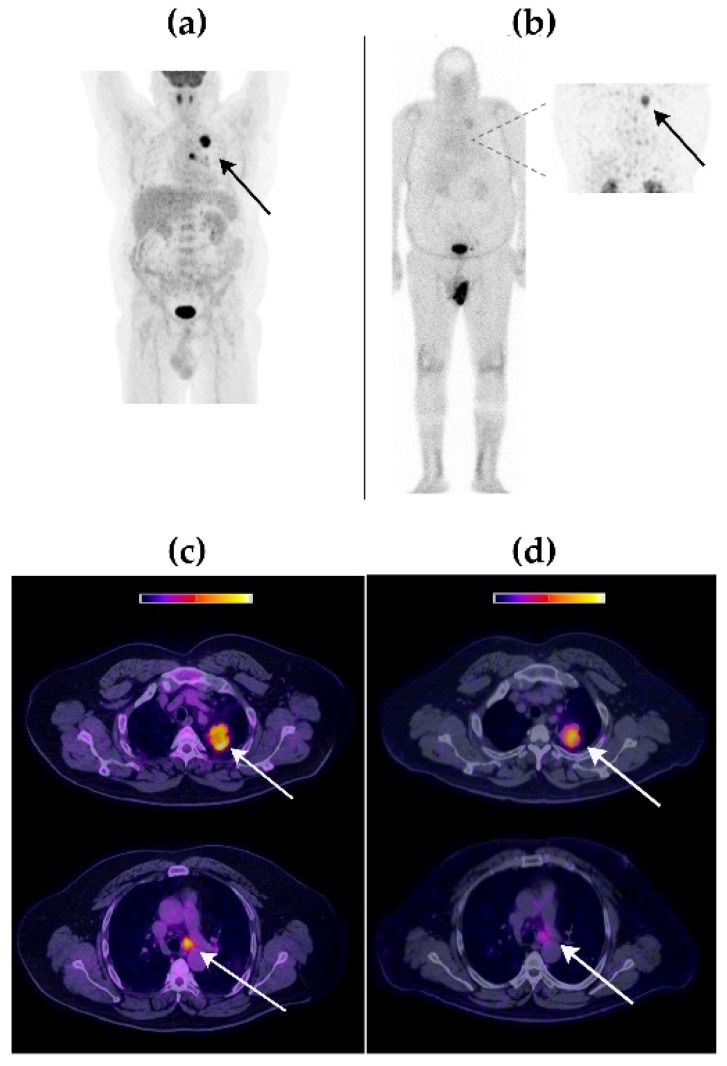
Patient with left lung adenocarcinoma (Patient 2). (a) Maximum-intensity projection [^18^F]FDG PET image at 1 h p.i. (b) [^99m^Tc]Tc-iFAP whole-body planar scintigraphy in anterior projection. Inset: thoracic SPECT at 3 h p.i. (c) [^18^F]FDG PET/CT axial sections. (d) [^99m^Tc]Tc-iFAP SPECT/CT axial sections. Solid lesion in the apicoposterior segment of the left upper lobe with irregular edges, which contacts the mediastinal pleura. Mediastinal adenopathy at subaortic level 5. The uptake of both radiopharmaceuticals was concordant in all tumor lesions.
